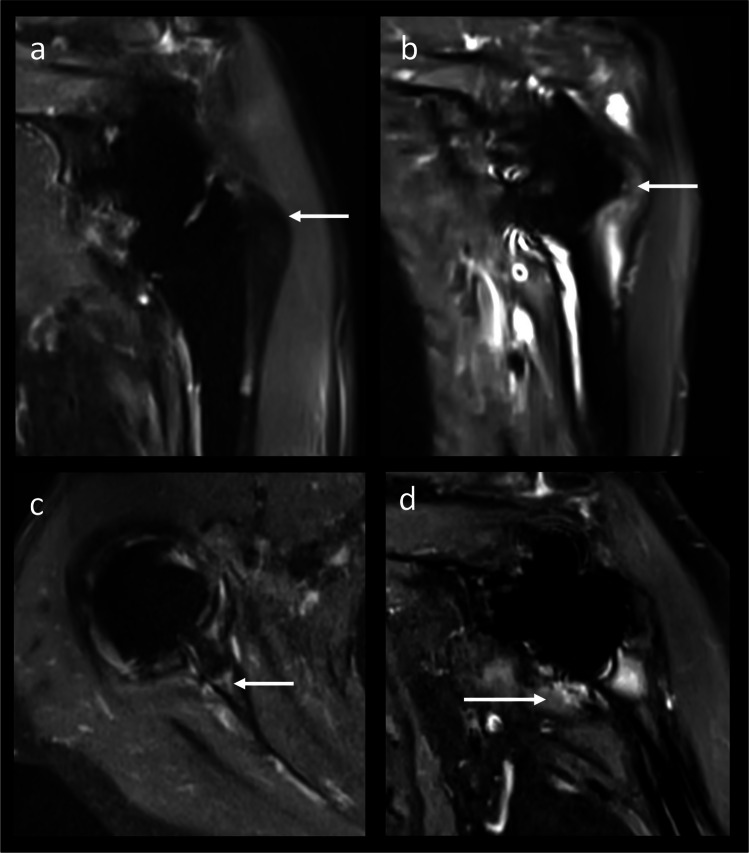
MRI findings associated with inferior clinical outcome measures 1 year after rTSA. In contrast to no BME at the greater tubercle (Gruen zone 1; a 70 years, male), subjects with severe BME at the greater tubercle (b 75 years, female) and those with BME in the medial glenoid (Molé zone 3; c 80 years, female) had inferior clinical outcomes. Synovitis (d 69 years, female) was a frequent finding, but was associated with inferior clinical outcomes. BME, bone marrow edema; rTSA, reverse total shoulder arthroplasty
